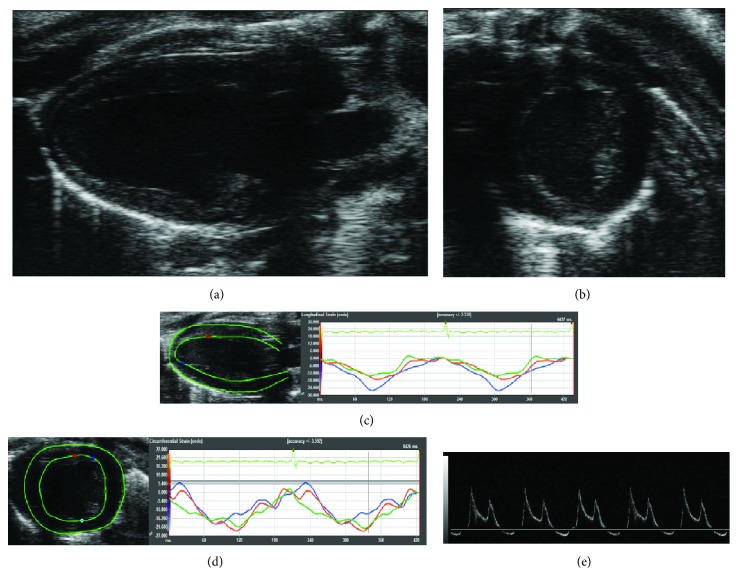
Cardiac ultrasound scan. (a) Parasternal long axis view of the left ventricle. (b) Parasternal short axis view of the left ventricle. (c) Analysis of the longitudinal and radial strain/strain rate. (d) Analysis of the circumferential and radial strain/strain rate. (e) Parasternal 4-chamber view with PW-Doppler for the evaluation of E/A parameter.
